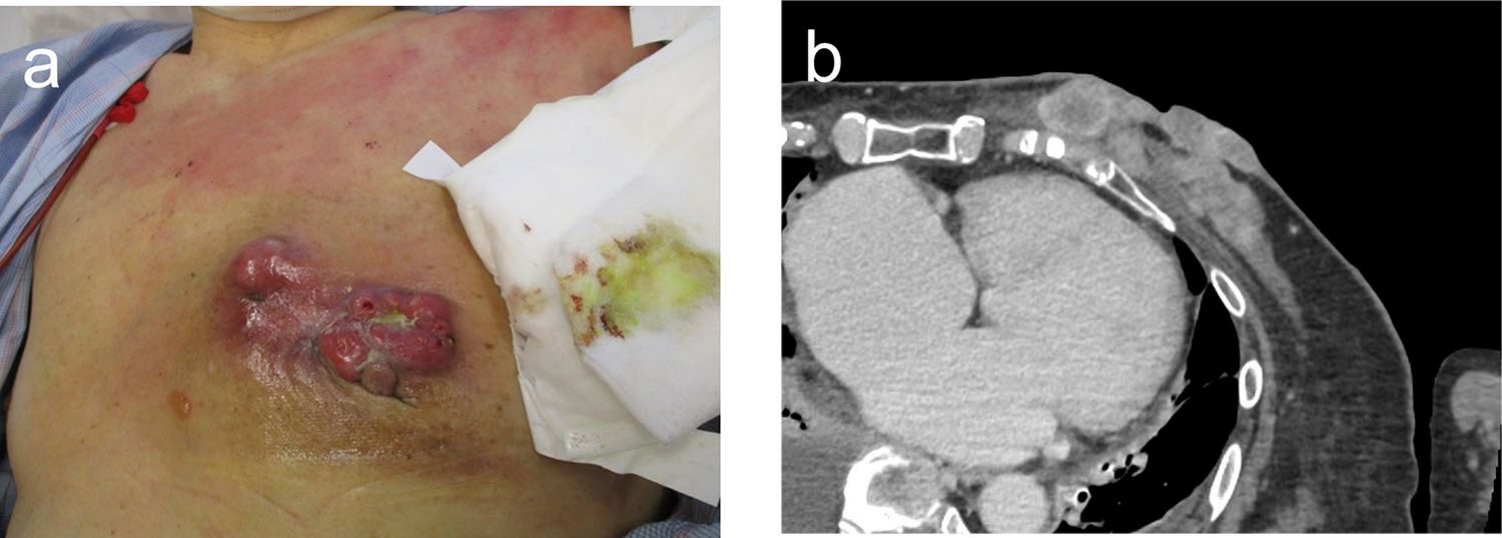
Response of the breast mass to radiation therapy. a Regression of the mass at four months post-treatment, observed on physical examination. b CT scan at 4 months following the completion of radiation therapy
